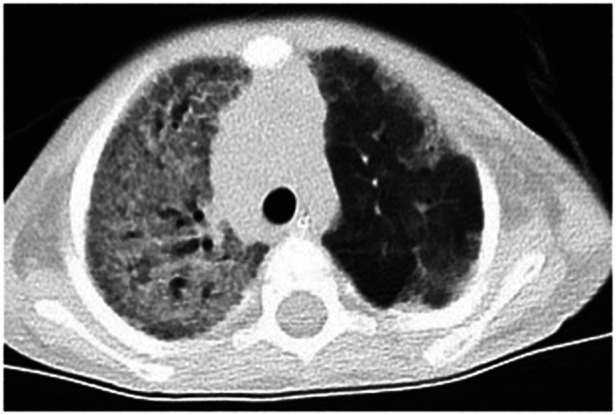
The chest-CT on the 14th day after hospitalization showed both lungs decreased translucency, extensive parenchymal opacities, and ground glass change. Some showed grid change and slightly dilated bronchus throughout the course.
